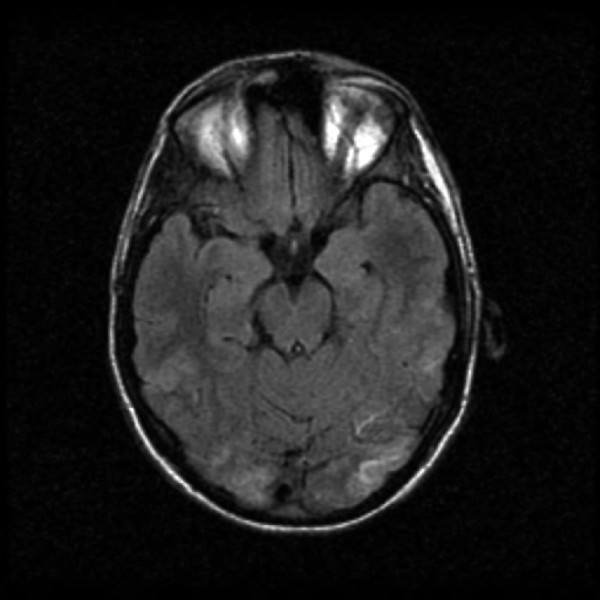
A T2-weighted MRI image of his head shows bilateral, slightly asymmetric, multifocal T2 hyperintensities in the posterior temporo-occipital subcortical white matter. There were no DWI (image not shown) signal abnormalities to suggest ischemia. These findings are most consistent with vasogenic edema. Post-gadolinium images (image not shown) demonstrated no abnormal enhancement.
